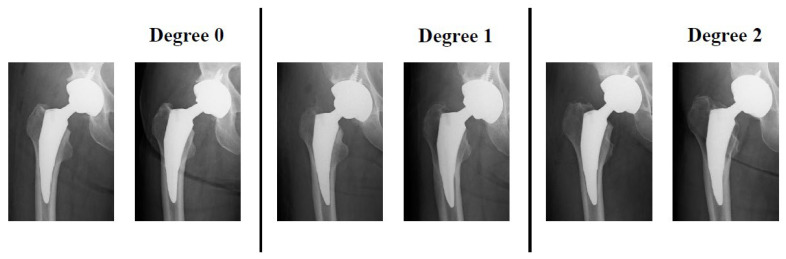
Representative radiographic image for each stress shielding degree. Left panel, immediately after surgery; Right panel, 2 years after surgery.
